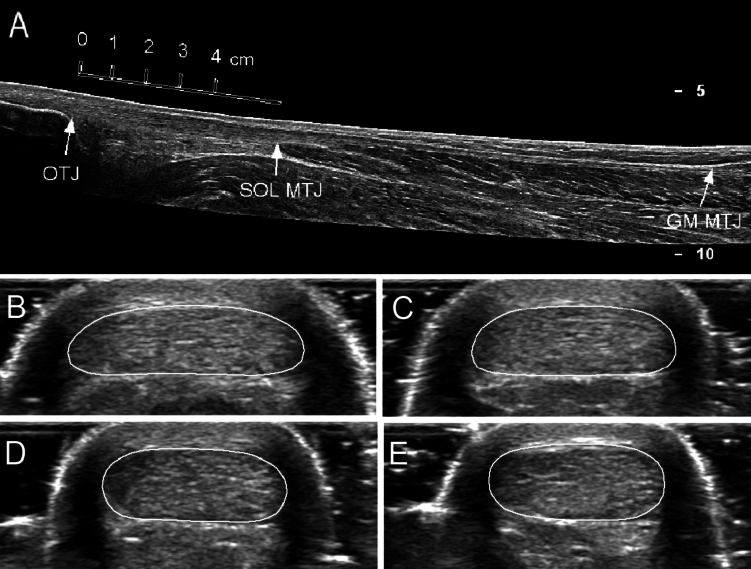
Representative extended field of view (EFOV) ultrasound image of the GM tendon length from distal GM myotendinous junction (GM MTJ) to osteotendinous junction (OTJ), along with the ‘free’ tendon length from the OTJ to the distal soleus MTJ (SOL MTJ), where CSA images were taken (A); and ultrasound transverse CSA images of the Achilles tendon at 1 (B), 2 (C), 3 (D) and 4 (E) cm proximal to the OTJ, in which the tendon CSA perimeter has been highlighted
